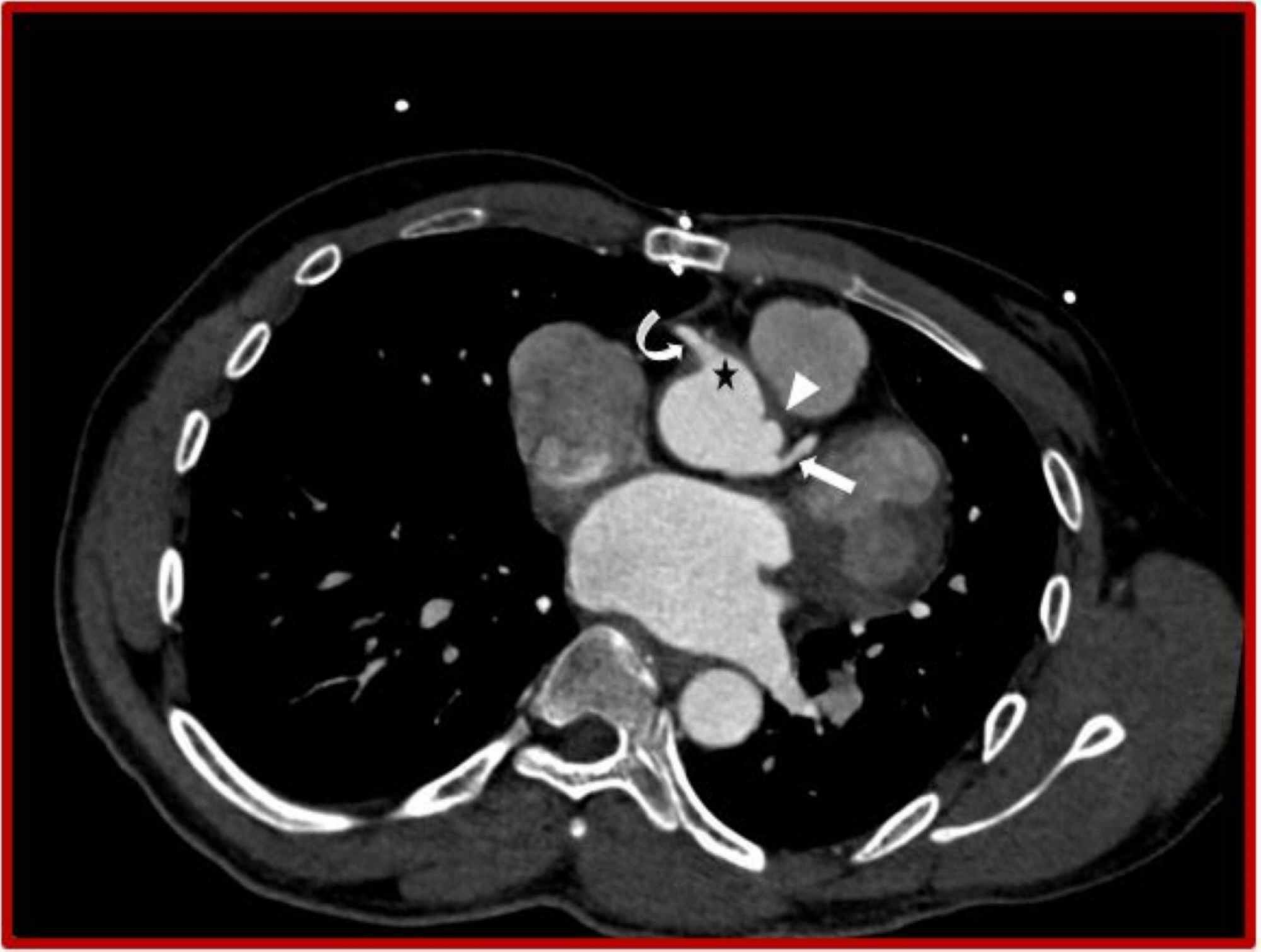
Cardiac CT (Axial view) demonstrates right (asterisk) and left (arrowhead) sinus of Valsalva aneurysms with right (curved arrow) and left (straight arrow) coronary arteries originating from them. CT: Computed tomography.
